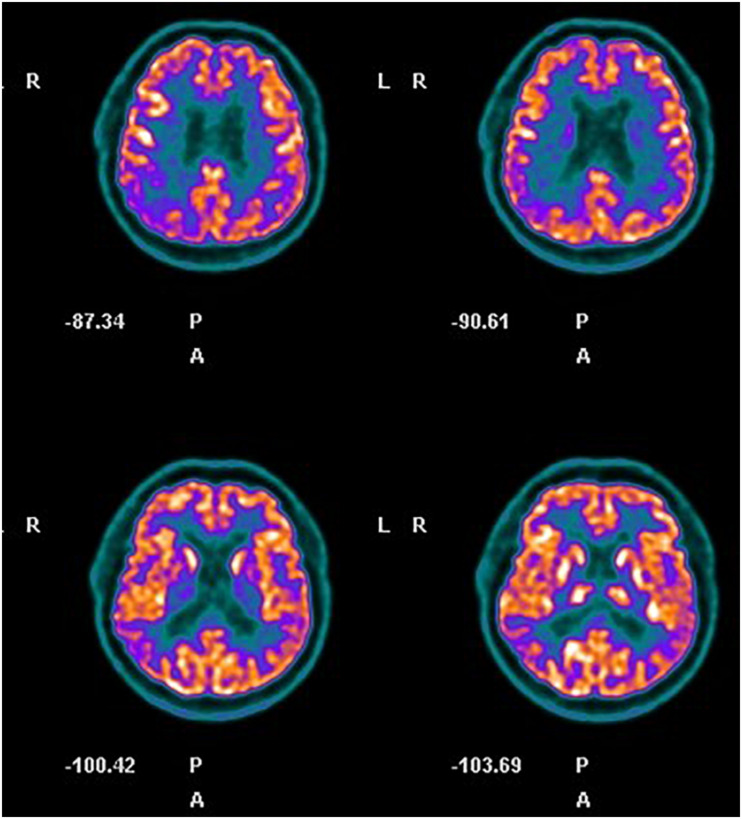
Case 2, cerebral FDG-PET. Hypometabolism in right parietal and temporal areas and at the anterior cingulate cortex is evident.
